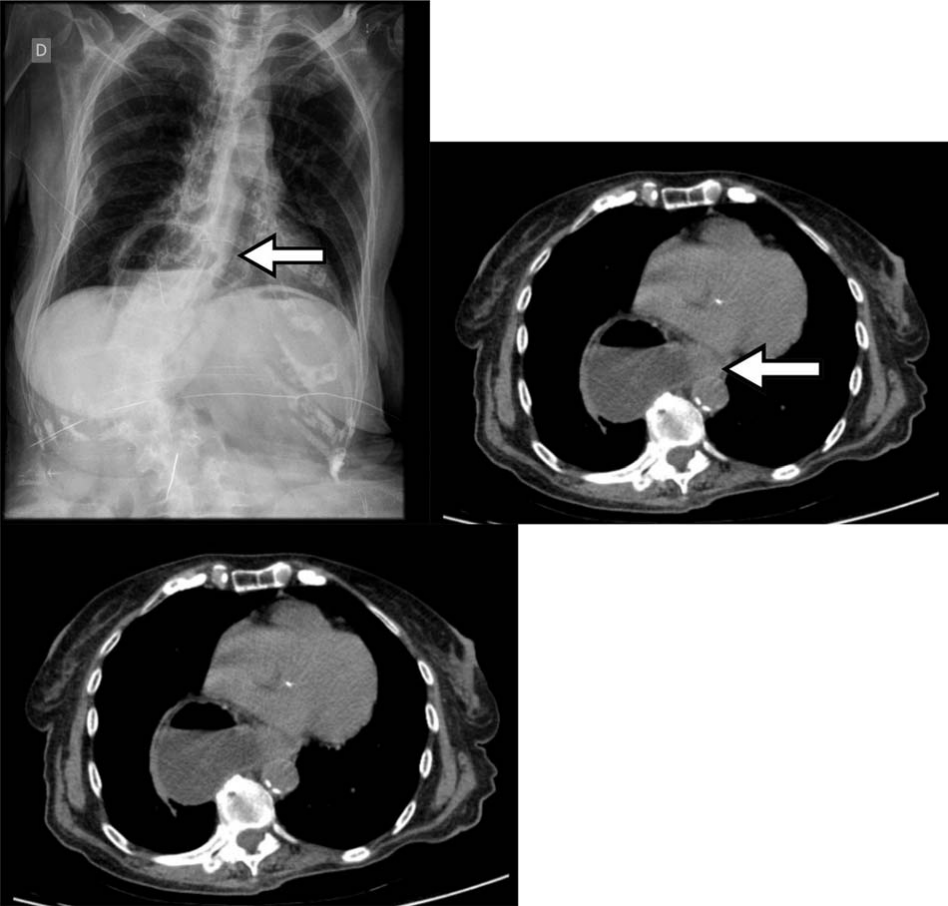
Chest X-ray with gas distension and the air-fluid level above the diaphragmatic domes and contrast-enhanced abdominal CT showing gastric volvulus in hiatal hernia.
